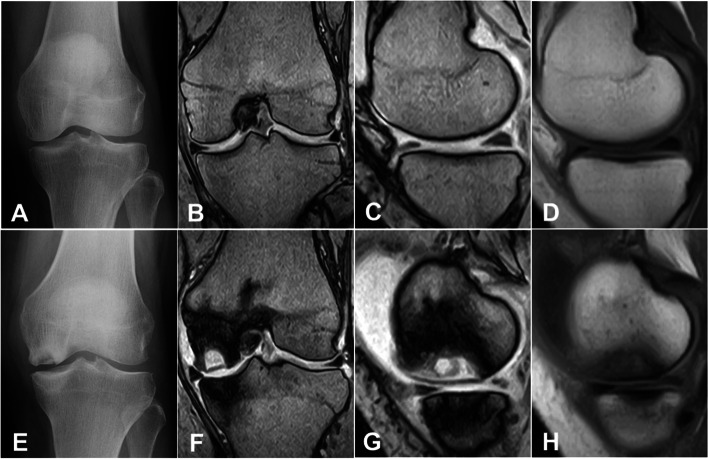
62-year old woman. Preoperatively, the radiogram (A) showed grade-1 OA. The images taken with the 0.3-T MRI (B and C: T2*, D: T1) did not show any findings of osteonecrosis or bone marrow edema (BME). At 3 months after the meniscectomy, the radiograms (E) indicated stage-3 osteonecrosis in the medial femoral condyle. MR images (F and G: T2*, H: T1) showed a necrotic lesion, which was surrounded by an osteosclerotic zone and a wide BME area. The resolution of the MR images was acceptable to measure the meniscus location on the joint line
